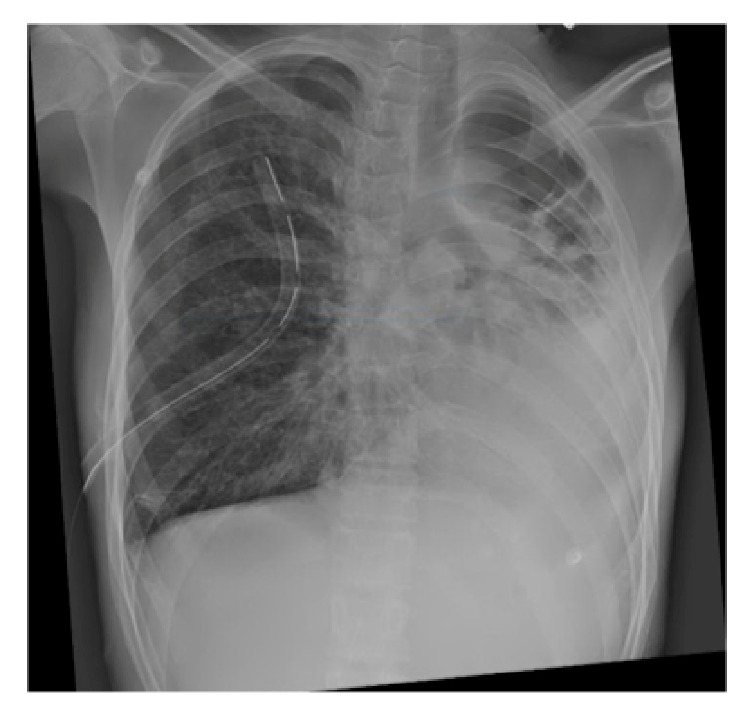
Chest X-ray after insertion of a new chest tube and resolution of the right-sided pneumothorax.
